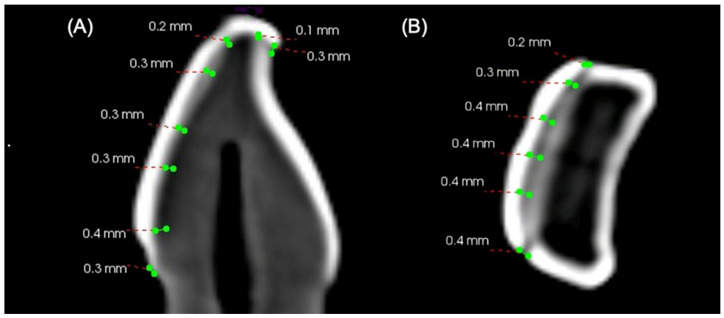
Representative CBCT sectional images representing measurements for internal gap and marginal discrepancy at incisal edge and cervical edge: (A) sagittal view and (B) axial view.
